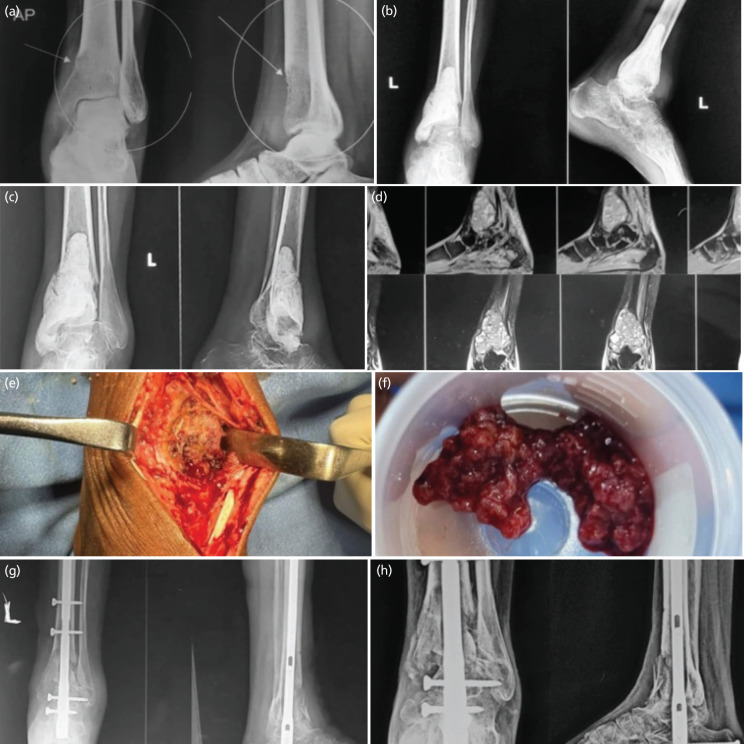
(a) Radiograph showing a lytic lesion in the distal tibia with subarticular extension. (b) Post-operative radiograph after curettage and bone cementation. (c) One year post-operative radiograph with signs of tumour recurrence. (d) Magnetic Resonance Imaging confirming the recurrence of tumour with soft tissue extension. (e) Intra-operative image of the tumour. (f) Intra-operative image of the curetted tumour. (g) Post-operative radiograph showing ankle arthrodesis using a retrograde locked intramedullary nail with autologous iliac crest bone graft. (h) Six months post-operative radiograph.
